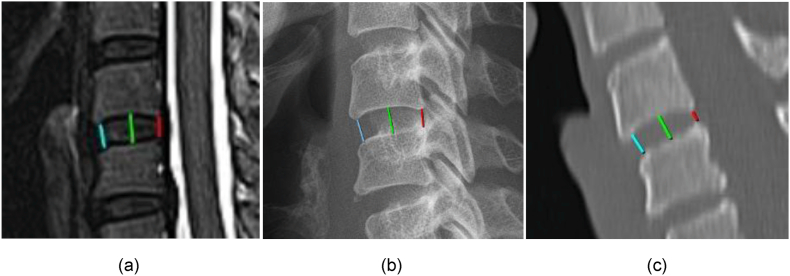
Example of C4-C5 mid disc height (green line), anterior disc height (blue line), and posterior disc height (red line) measured in the same patient; (a) MRI; (b) X-ray; (c) CT.
